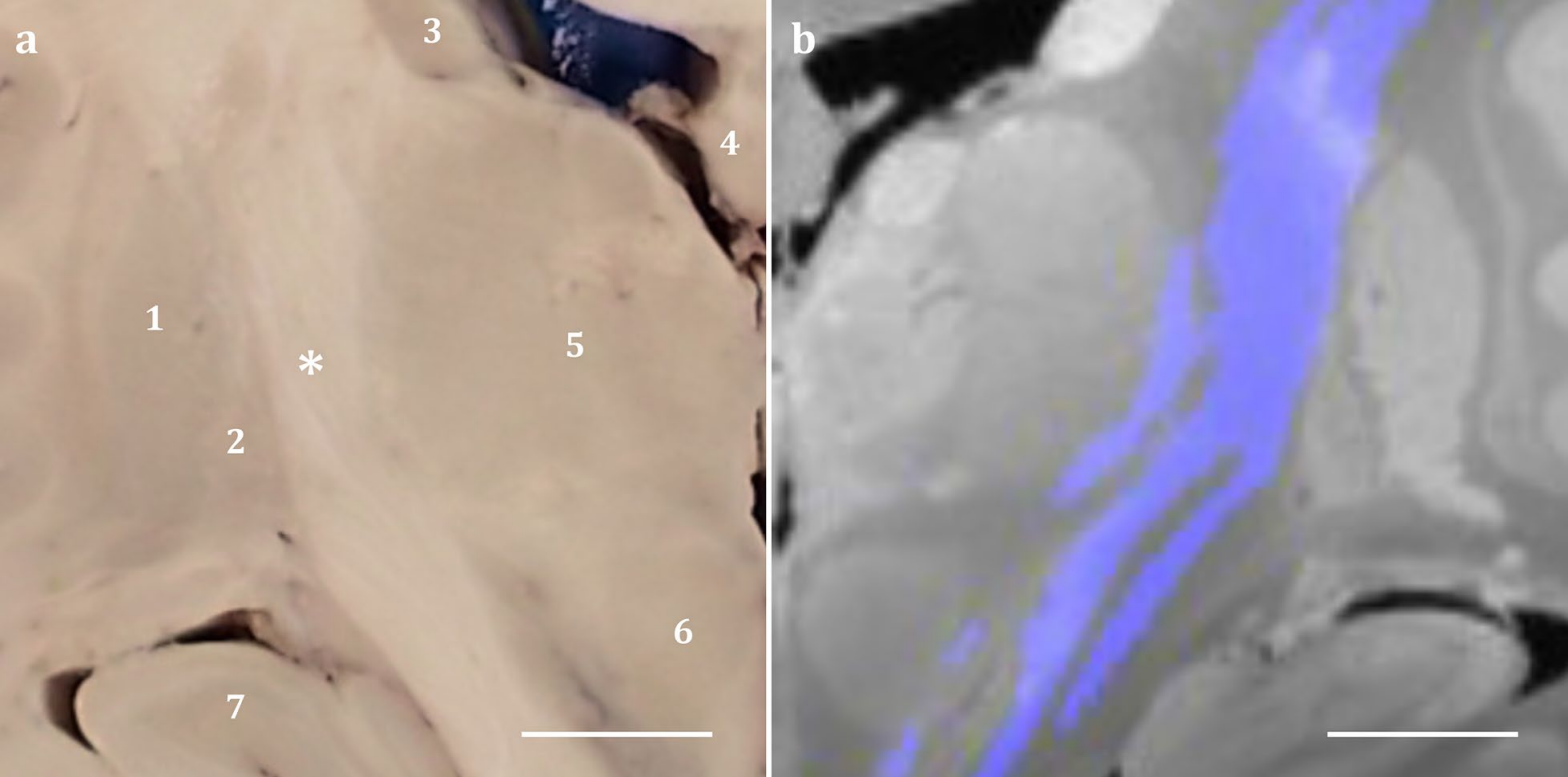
Tractography guided identification of the corticospinal tract. The slice (a) that best represents the corticospinal tract (purple) was identified with tractography (b mirrored MR image). Annotations—1: putamen, 2: external globus pallidus, 3: caudate, 4: body of fornix, 5: thalamus, 6: red nucleus, 7: hippocampus, *posterior limb of internal capsule. Scale bar = 1 cm
